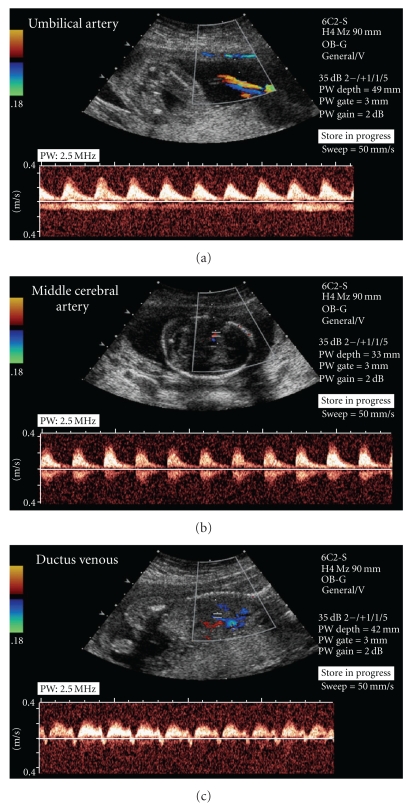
Doppler velocimetry of fetal vessels. (a) depicts a normal umbilical artery flow waveform. (b) depicts a normal waveform of the middle cerebral artery, and (c) depicts an abnormal ductus venosus waveform, showing a reversed “a” wave.
